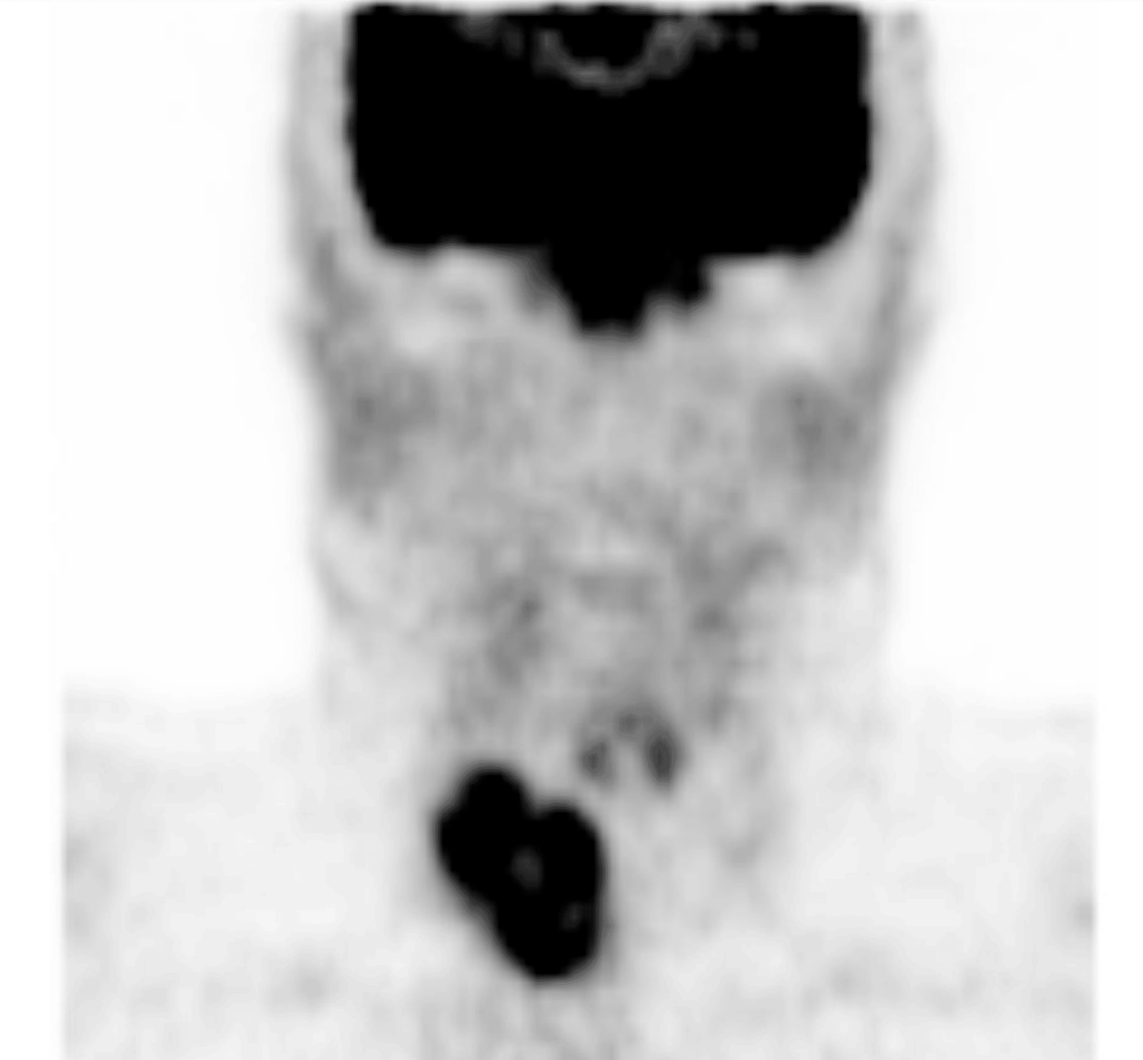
Fluorodeoxyglucose (FDG)-PET/CT demonstrating a markedly FDG-avid mass in the right thyroid lobe, measuring approximately 4.2 cm with a maximum standardized uptake value (SUVmax) of 9.3.
